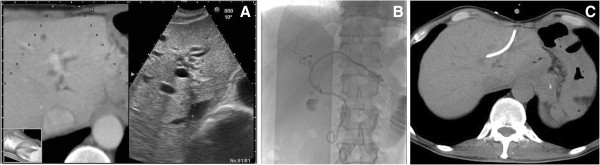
A 55-year-old man with jaundice due to peritonitis carcinomatosa from gastric cancer. A: The virtual CT sonographic image (left) displayed with the corresponding B-mode sonogram (right) shows the dilated bile duct (B3: arrow). The virtual image shows that the stomach cavity (arrow head) is close to the lateral segment of the liver. However, a B-mode sonogram cannot show the stomach cavity in the same view. The virtual image helps to determine the safe puncture route. B: The radiograph shows successful internal-external bile duct drainage using a 8.5 French drainage catheter. C: The CT image shows that the drainage catheter is inserted into the bile duct through the route planned before the procedure using the RVS system. CT: computed tomography.
